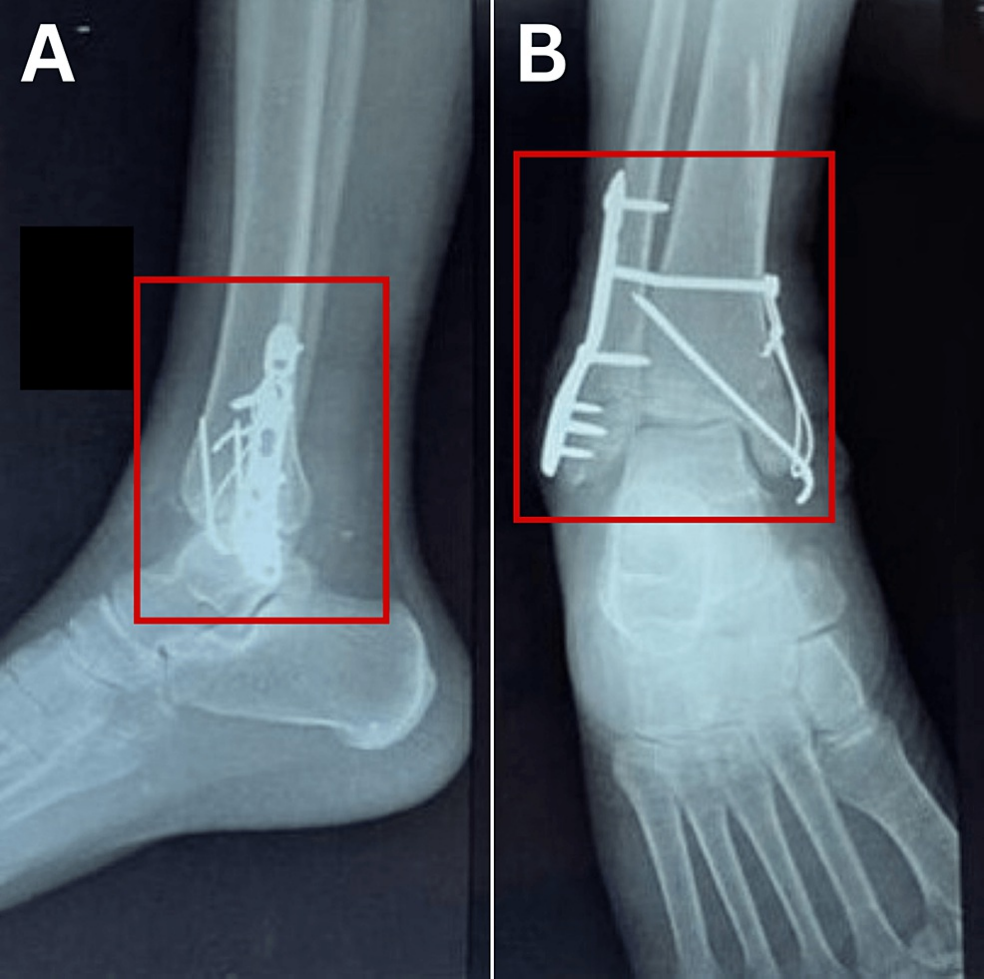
Lateral and anteroposterior (AP) views of the post-operative X-ray of the right ankle Figure 1A, 1B : Red-colored rectangle demonstrates the trimalleolar fracture, which was treated by open reduction and internal fixation (ORIF) along with a cannulated cancellous screw and rush nail in the lateral and anteroposterior views, respectively.
